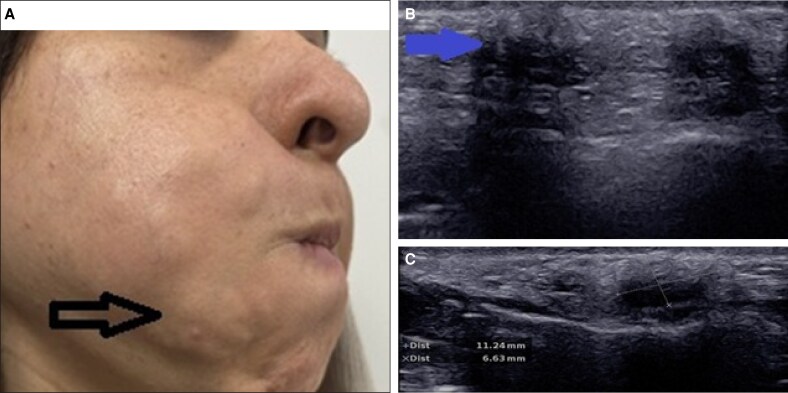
(A) A 36-year-old woman with a history of polycaprolactone (PCL) injection 2 years ago presents with multiple nonresolving nodular lesions in the right buccal region that developed 4 months after the injection. (B, C) Ultrasound images reveal multiple hypoechoic subcutaneous masses with echogenic foci on their surfaces (blue arrow in B), causing posterior shadowing, consistent with a PCL granulomas.
